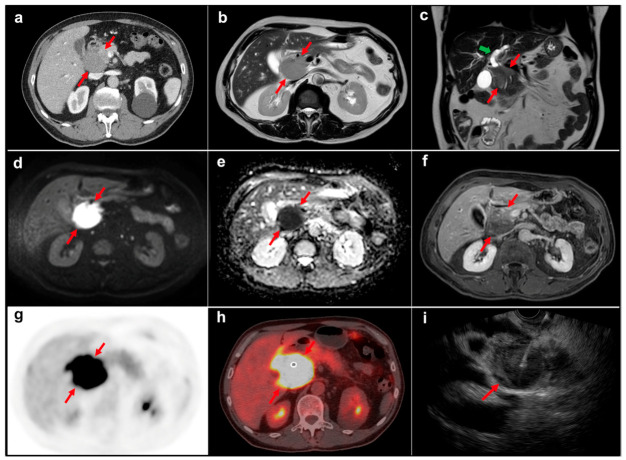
Pancreatic/peripancreatic lymphoma in a male patient presenting with obstructive jaundice. (a) Axial pancreatic-phase CT shows a homogeneous soft-tissue mass with mild enhancement in the pancreaticoduodenal groove, infiltrating the pancreatic head (red arrows). (b–f) Subsequent MRI shows a mildly hyperintense mass on axial and coronal T2WI (b,c, red arrows), without a capsule-like “halo” sign, infiltrating the intrapancreatic CBD and causing upstream biliary dilatation (c, green arrow). The mass shows marked restricted diffusion on DWI (b = 800 s/mm^2^) with corresponding low ADC values (d,e, red arrows), and mild enhancement on portal-phase DCE-MRI, similar to that observed on CT (f, red arrows). (g,h) ^18^F-FDG PET/CT demonstrates marked hypermetabolism of the mass, with a maximum standard uptake value (SUVmax) of 28 g/mL (red arrows). This constellation of findings favours a lymphoma-related bulky mass over other differential diagnoses such as focal AIP or PDAC. (i) Endoscopic ultrasound with fine-needle biopsy (EUS-FNB) of the mass (red arrow) and bone marrow biopsy were performed, with a final diagnosis of Burkitt lymphoma.
